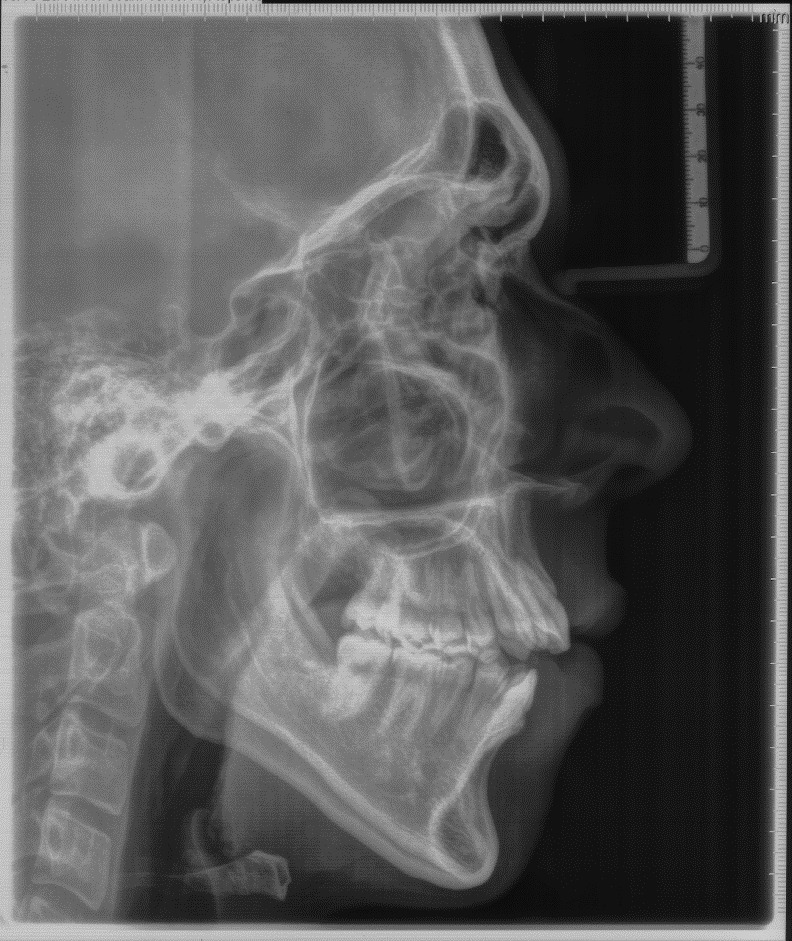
Pre-treatment lateral cephalometric radiograph.
